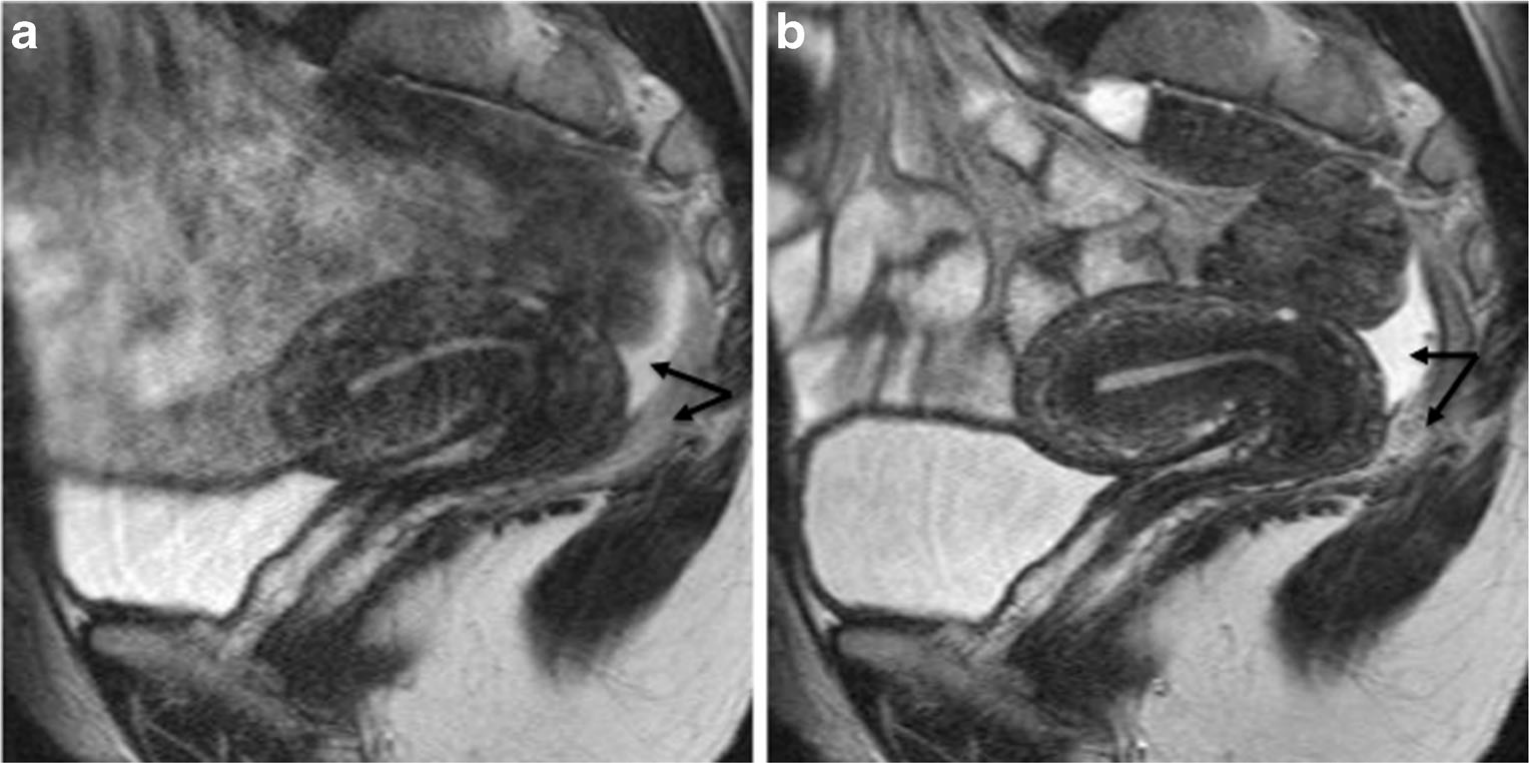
Sagittal 2D T2-weighted MR images performed at 1.5 Tesla showing the benefits of anti-peristaltic agents on image quality. Imaging performed in the same patient before (a) and after (b) administration of glucagon demonstrating a dramatic improvement in image quality. Note the presence of pelvic fluid in the pouch of Douglas underlining a clear demarcation between peritoneal and posterior subperitoneal compartments (double arrow) (reprinted with permission - Bazot M. Ed. Lavoisier-Paris 2016)
